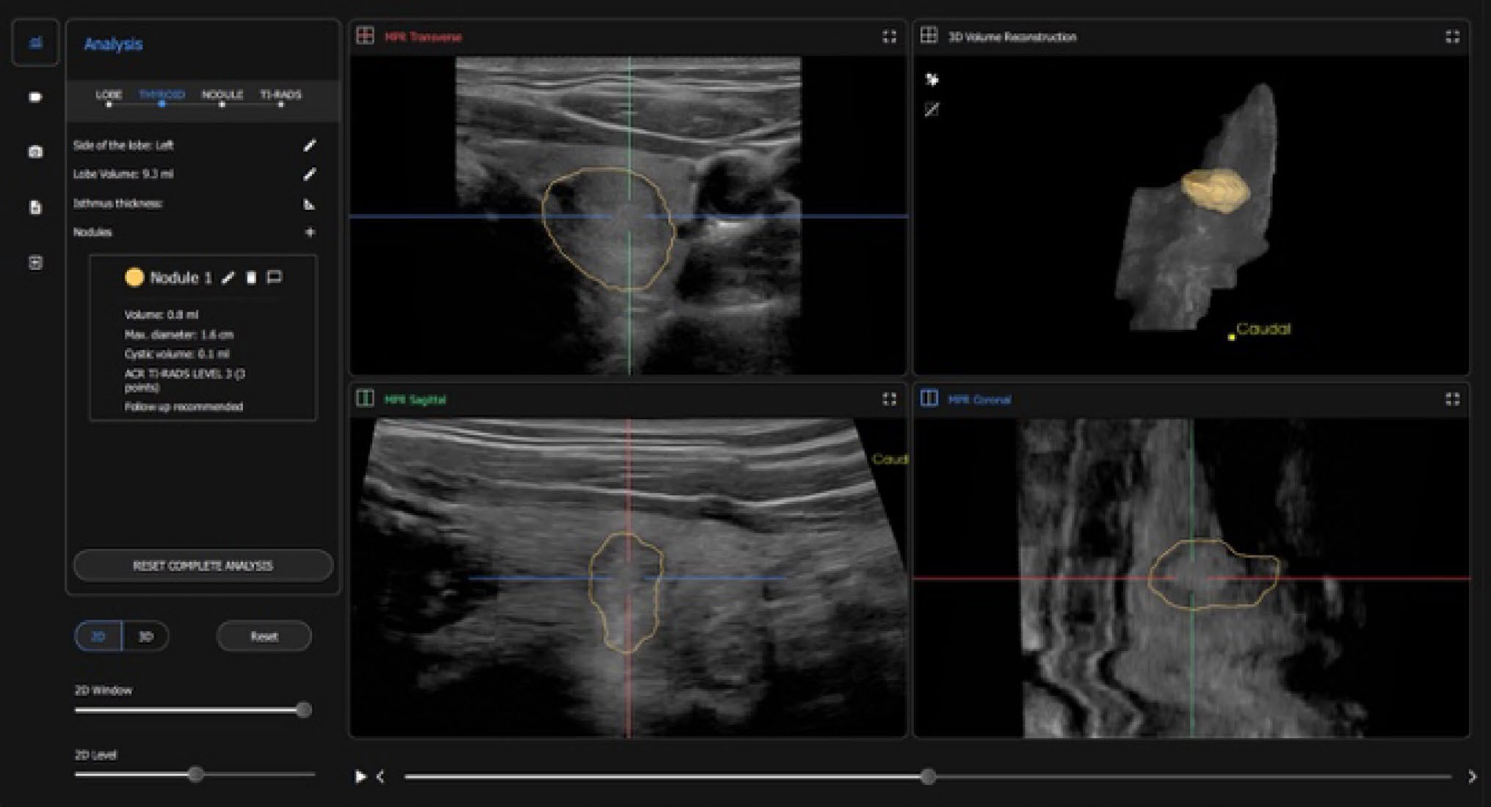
Example of an AI-assisted thyroid ultrasound examination result: 3D model of the thyroid lobe with lobe volume, detected nodules, nodule measurements, nodule volume and ACR TI-RADS assessment, as well as a recommendation for further courses of action
